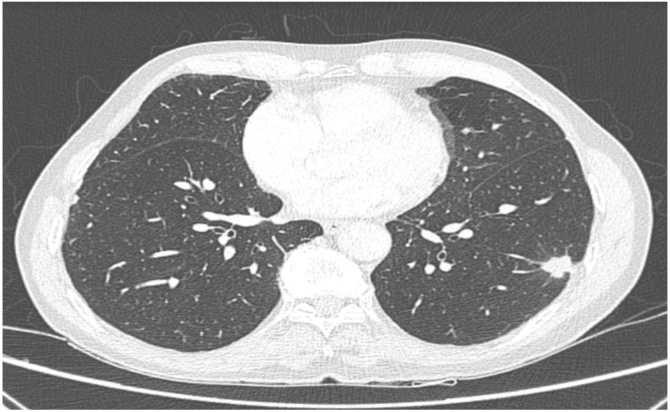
Computed tomography of the chest. There are bilateral spiculated pulmonary nodules, with the largest occurring in the superior segment of the left lower lobe, at 1.5 cm.
